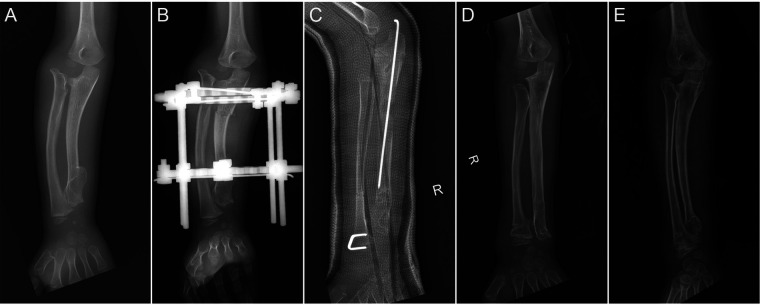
The preoperative and postoperative radiographical results of case 3. (A) The preoperative radiograph; (B) postoperative day 1 radiograph; (C) 1-month post-external fixator removement radiograph; (D) 3-year postoperative radiograph; and (E) 5-year postoperative radiograph.
